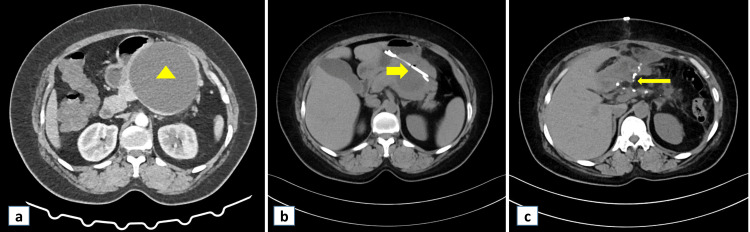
CT scan abdomen a) Arrowhead showing a single, well-defined hypodense cystic lesion of size 12.6 x 10 cm arising from the distal body and tail of the pancreas, b) the cyst size marginally decreased; broad arrow showing the cystogastrostomy stent in situ, c) follow-up CT; slender arrow pointing to no recurrence of the cyst
